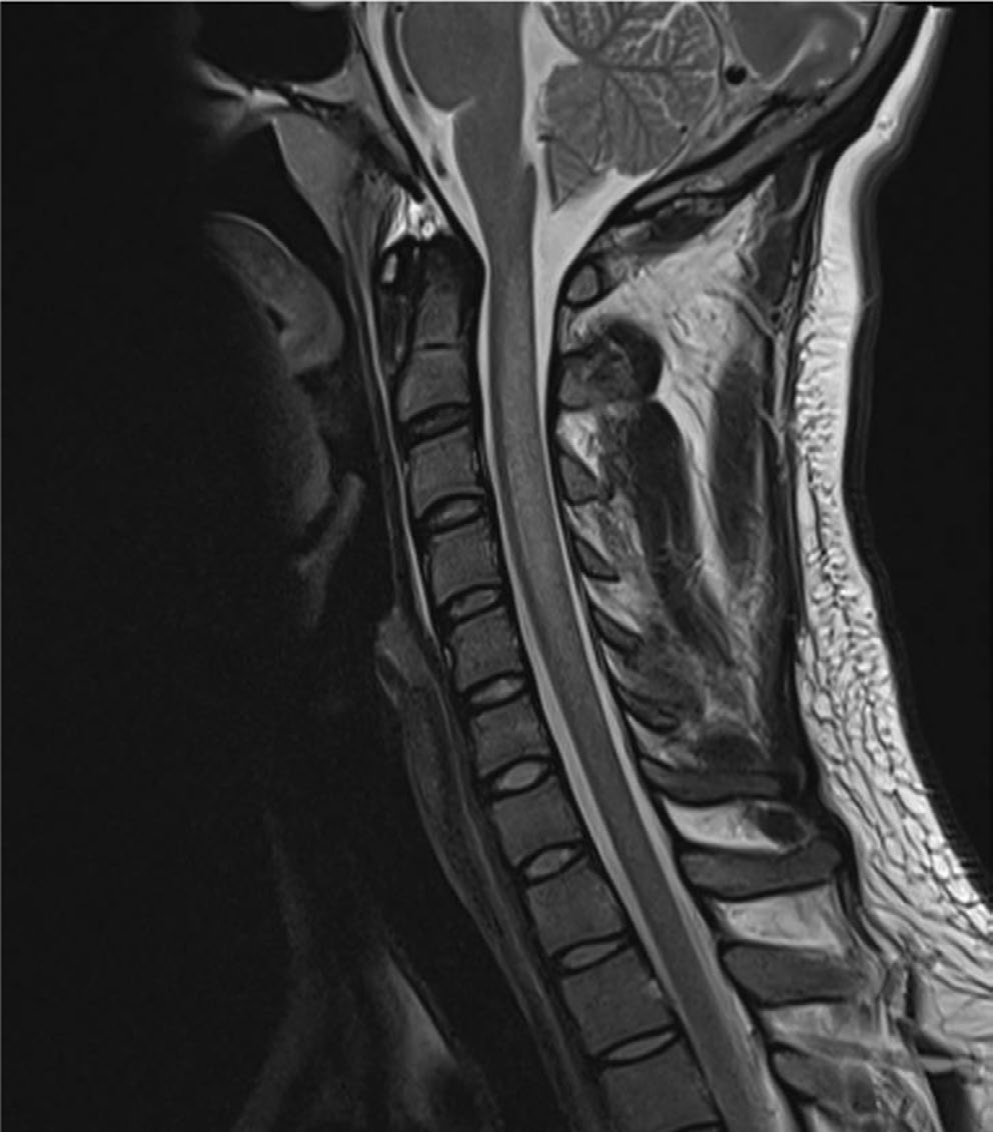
FIGURE 1 Cervical MRI. T2 hyperintensity is observed at cervical level, corresponding to patient 2.
